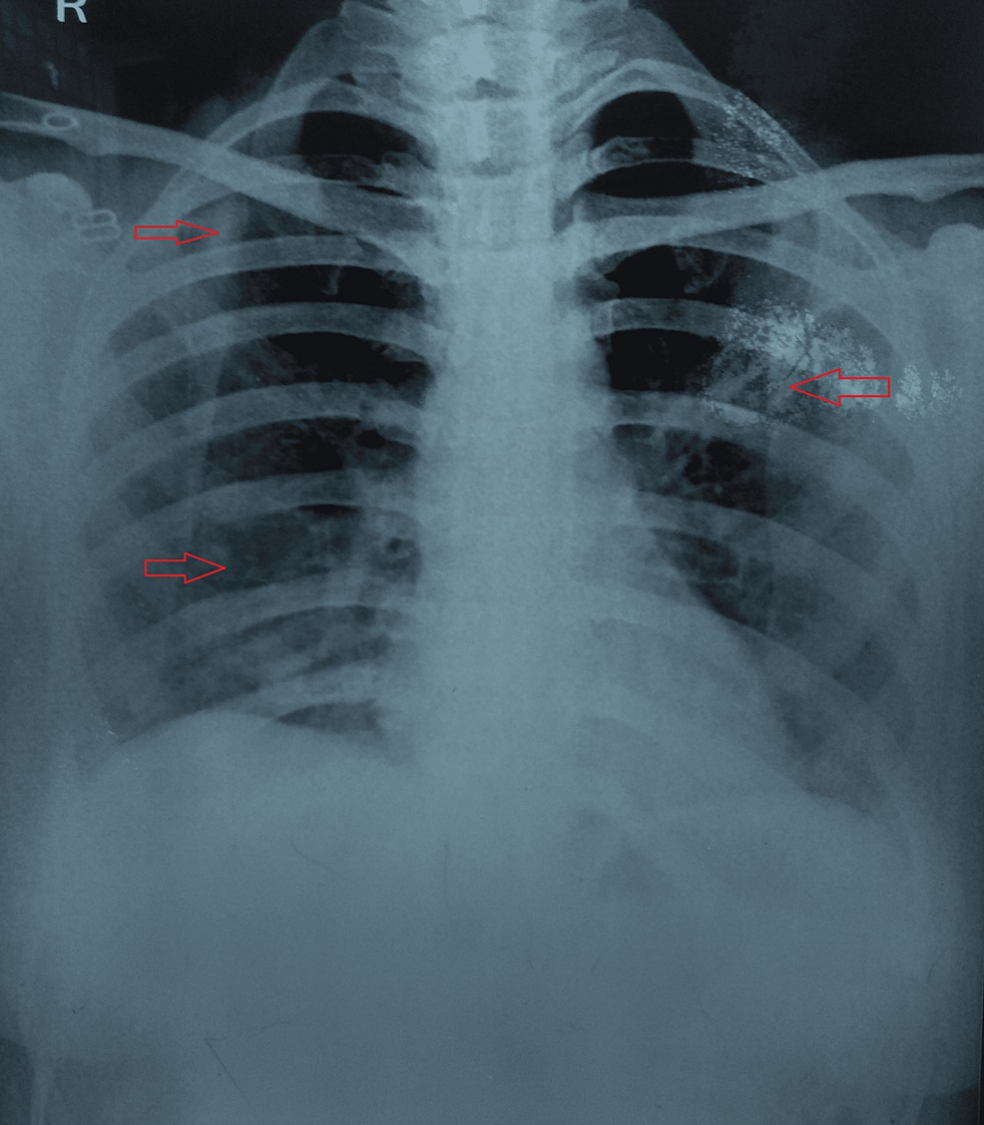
Plain chest radiograph suggestive of bilateral patchy consolidations
